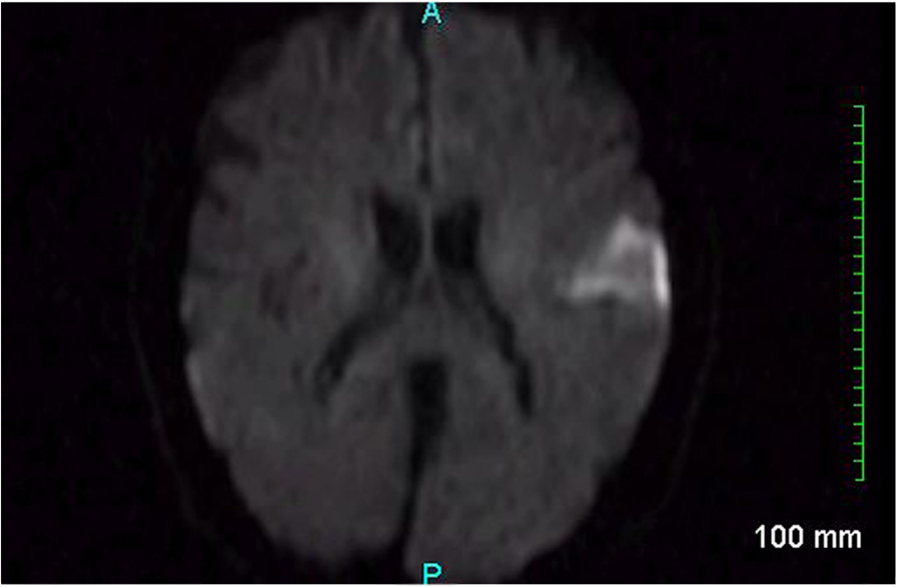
Diffusion-weighted magnetic resonance imaging demonstrating an acute ischemic infarct within the mid left temporal lobe.
